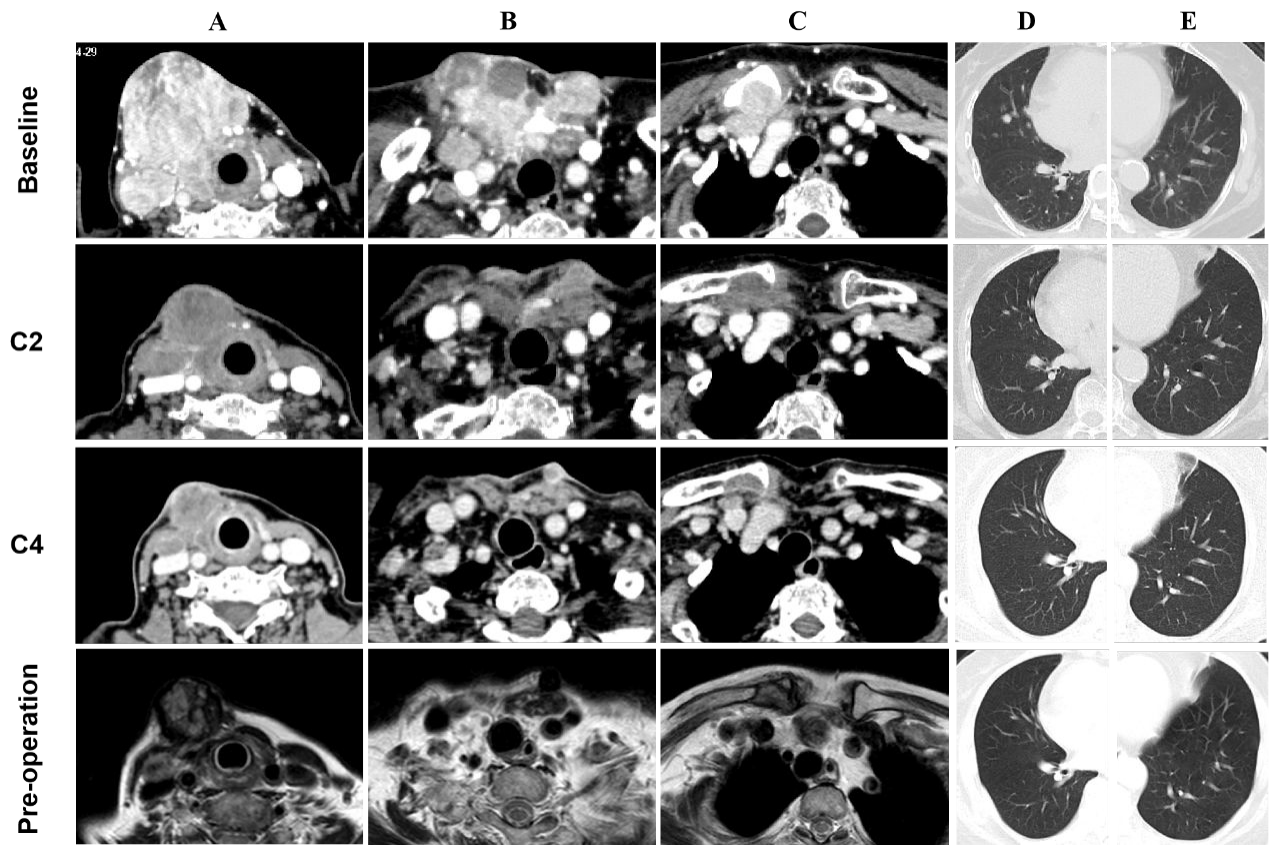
Imaging evaluation on tumor shrinkage following anlotinib therapy CT (Baseline, C2, C4); MRI (pre-operation). A: Recurrent tumor within thyroid bed. B: Recurrent tumor in the neck root in front of trachea. C: Clavicle and subclavian lymph nodes. D: Metastatic lesion in the right lung. E: Metastatic lesion in the left lung.
